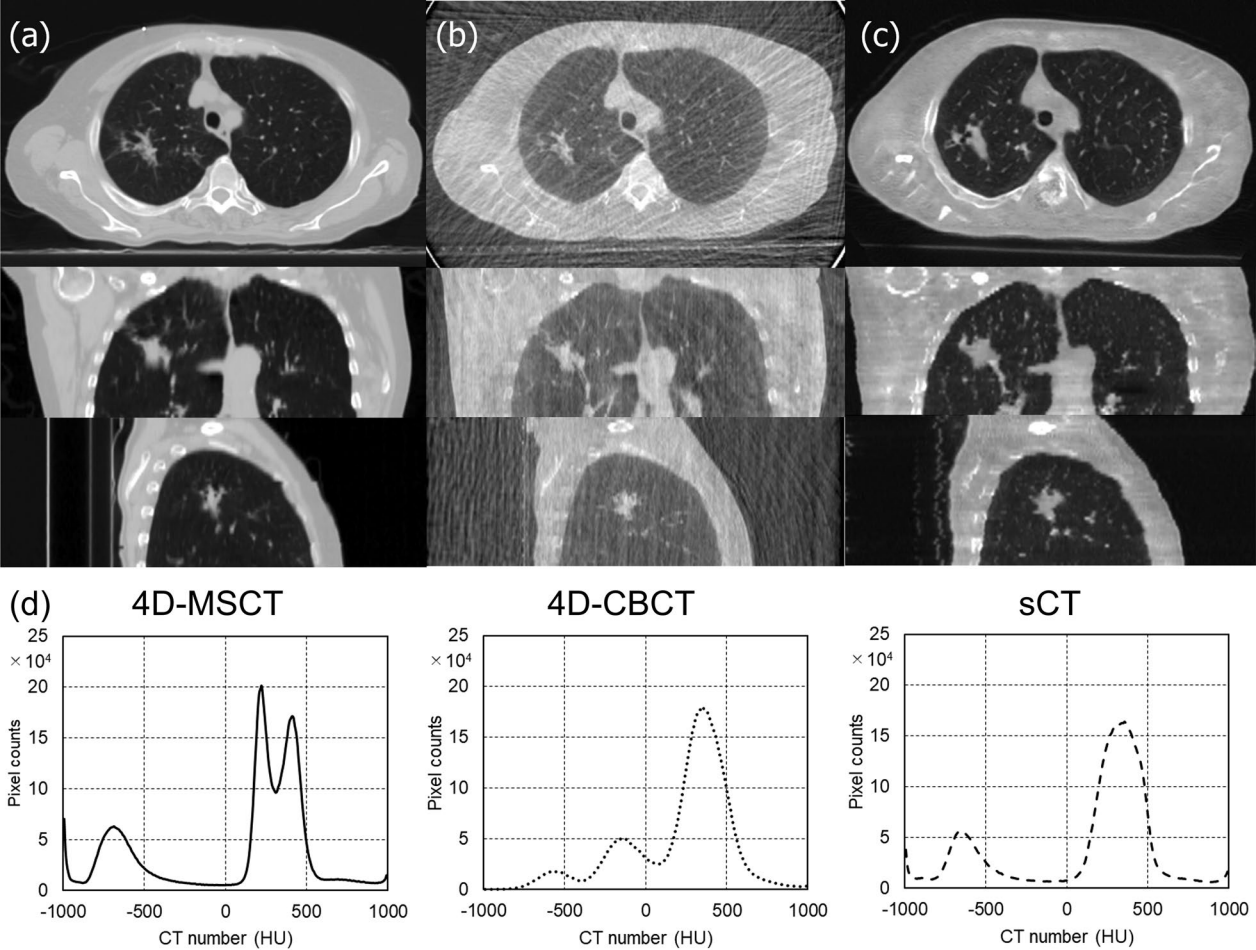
a 4D-MSCT, b 4D-CBCT, and c synthetic 4D-CBCT (sCT) images of the same patients in the corresponding axial, coronal, and sagittal directions. All images are shown with the same window width and levels. d Two-dimensional histograms of the axial direction in each image. The height of each histogram represents the count for the CT number
